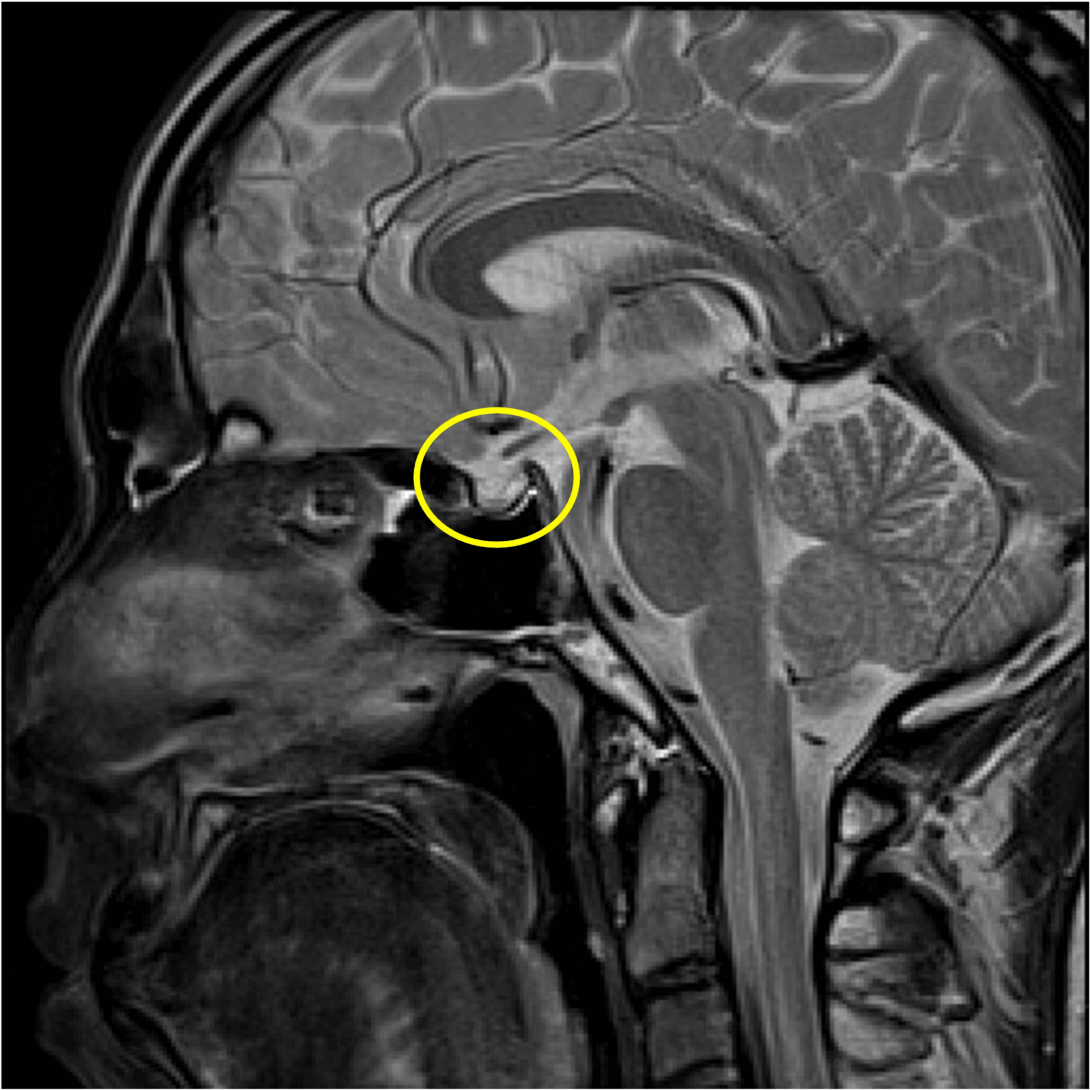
Contrast-enhanced MRI scan of the brain Sagittal T2-weighted MRI. The pituitary gland maintains a normal signal intensity and morphology without mass effect.
